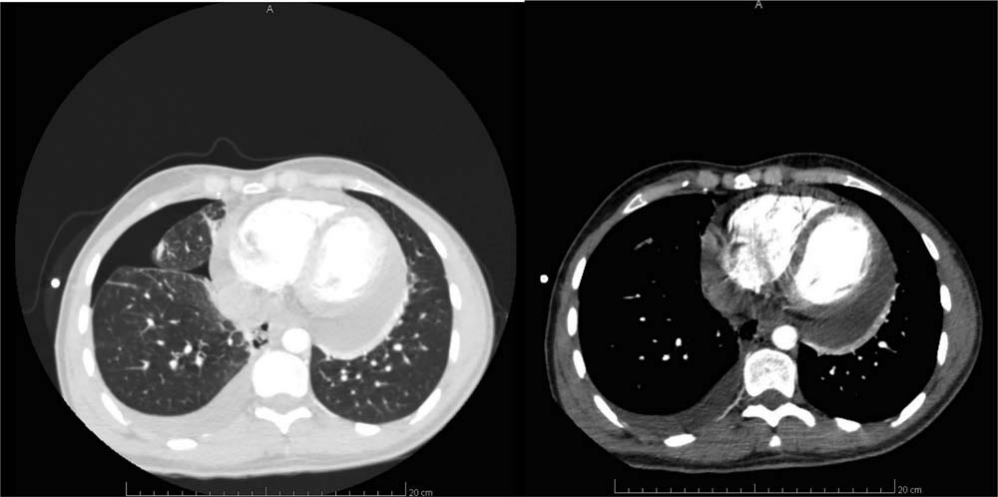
A and B. CT scan of the thorax: Right-sided pleural effusion with chest drain, pneumothorax and pneumomediastinum (left), pericardial fluid collection (right).
